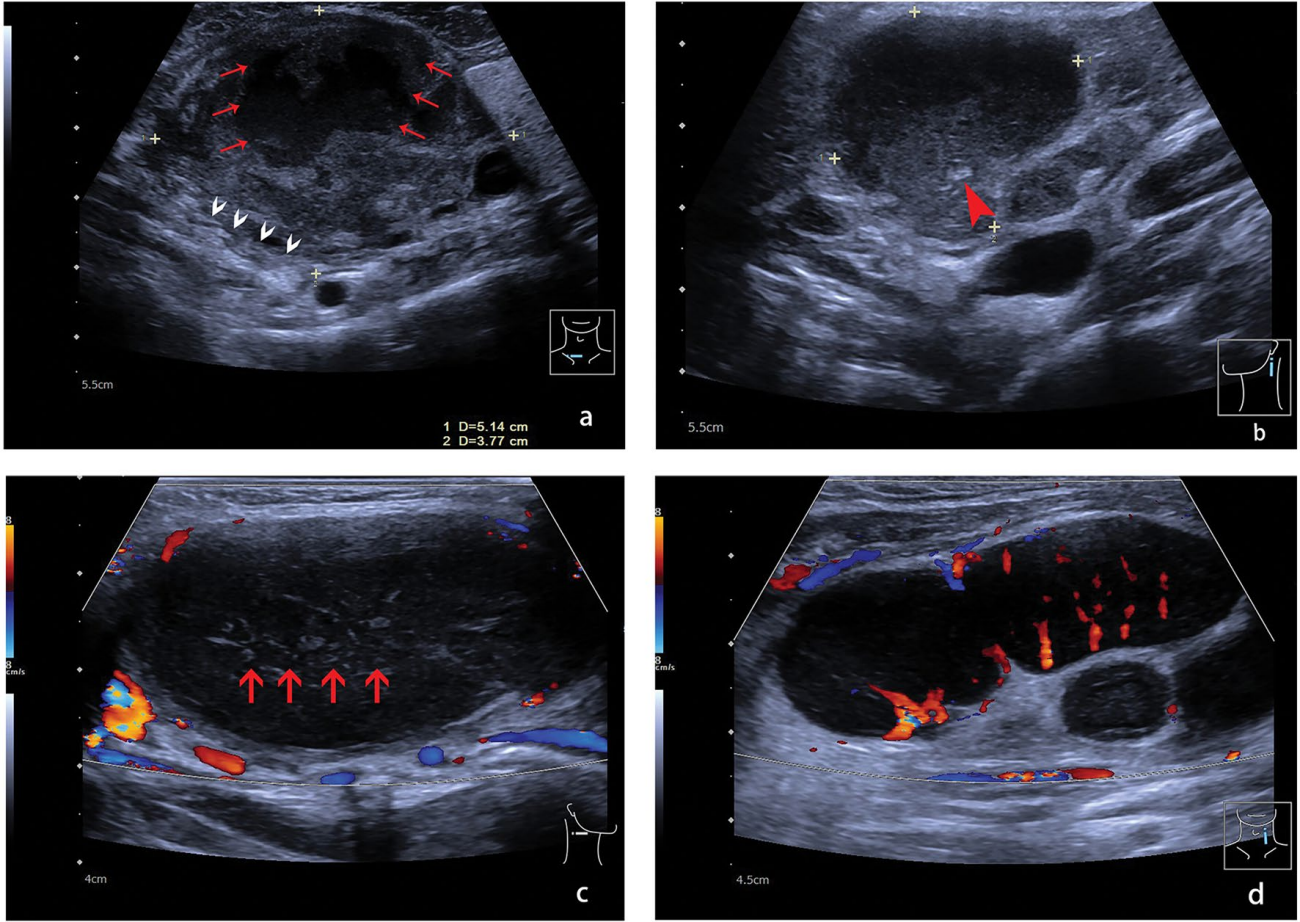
Ultrasound features of cervical lymph nodes. (a) Metastatic lymph node showing a long axis diameter (L) of 51.4 mm, short axis diameter (S) of 37.7 mm, L/S ratio < 2, absence of echogenic hilum, and a non-circumscribed margin (white arrows) with necrosis (red arrows); (b) Benign lymph node showing calcification (arrow); (c) Lymphoma showing grid-like echo (red arrows) and a peripheral vascular pattern; (d) Lymphoma showing a mixed vascular pattern.
